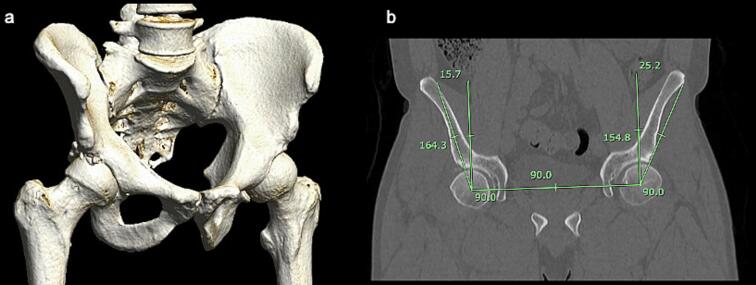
(a) CT 3D reconstruction showing irregularity in the anterior superior margin of the right acetabulum with adjacent bone fragments suggesting an apparent lack of acetabular coverage (antero-superior aspect) (b) quantification of center-edge angle at the coronal section where the irregularity of the superior-anterior margin of the acetabulum was more evident (25.2° left, 15.7° right).
